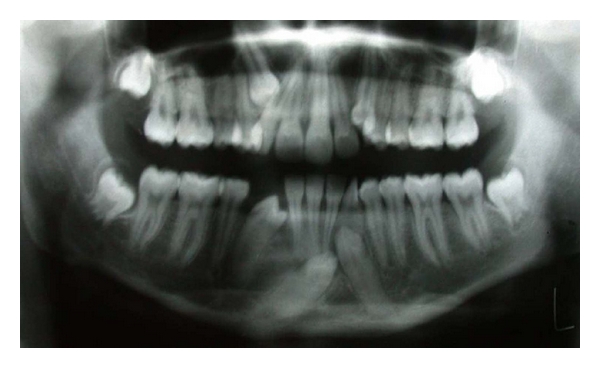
Panoramic radiograph showing 43 transmigrating towards left side.
